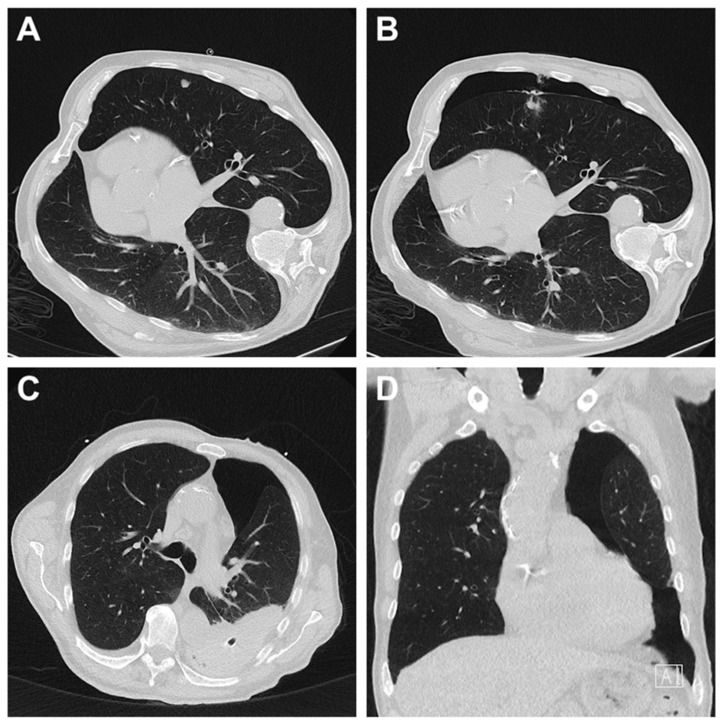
Hemopneumothorax after CT-guided biopsy of a pulmonary nodule in the left upper lobe. In a patient with renal cell carcinoma, CT-guided biopsy of a metastasis-suspected pulmonary nodule in the left upper lobe was performed (A). Immediately post-intervention, a pneumothorax measuring up to 4 cm was observed (B). 6 days after the intervention, despite the placement of a chest drain, a residual central pneumothorax with a rim width of up to 8 cm remained, accompanied by an organized left-sided hemothorax measuring up to 3 cm, containing multiple air inclusions (C,D). Due to the persistent hemothorax, video-assisted thoracic surgery for hematoma evacuation was performed.
